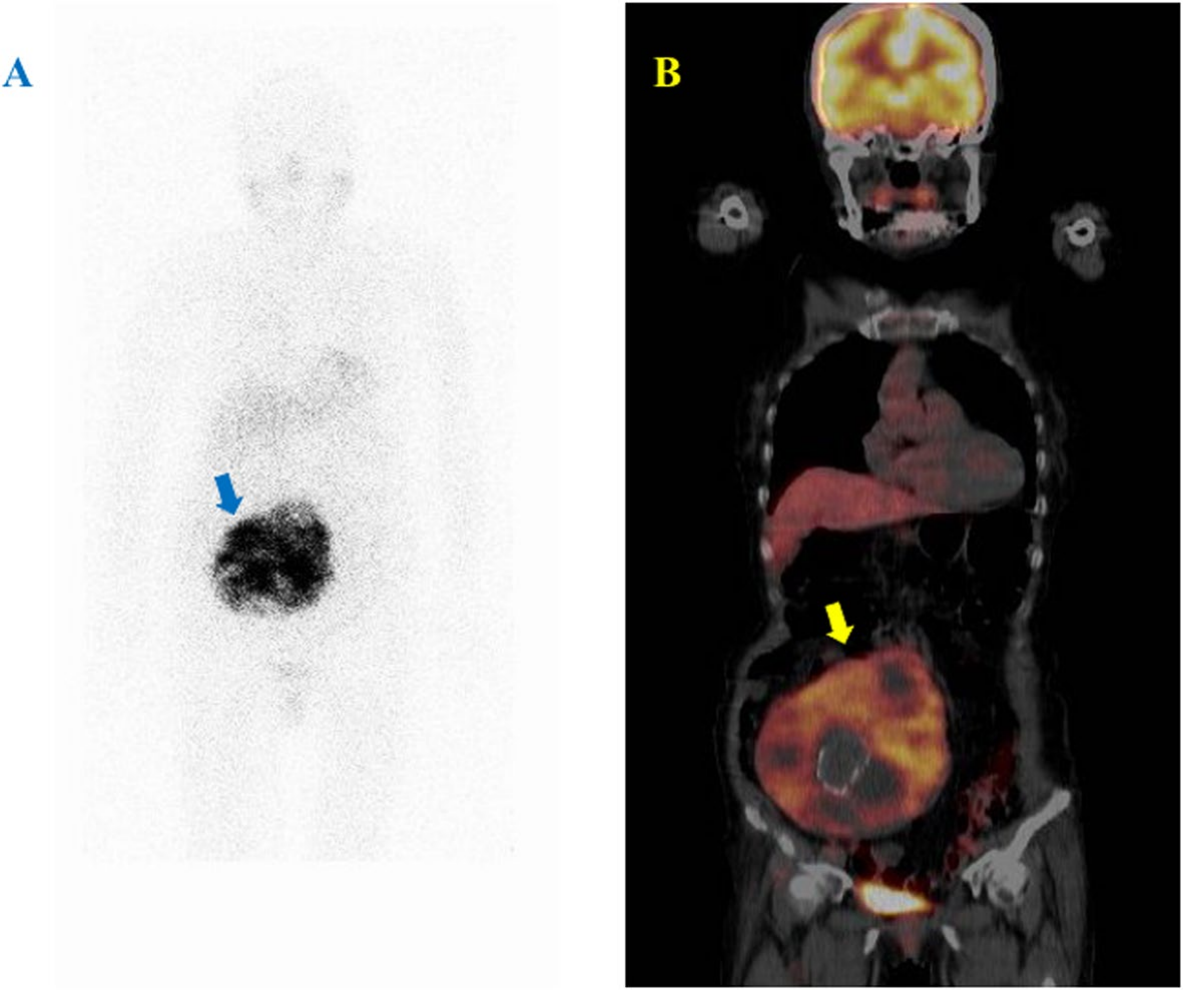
^18^F-FDG-PET and MIBG scintigraphy in paraganglioma without SDHB gene mutation. Sixty-one y.o. female of paraganglioma without SDHB gene mutation. (A) ^123^I-MIBG scintigraphy showed positive accumulation in right retroperitoneal to pelvic cavity. (B) ^18^F-FDG-PET showed SUVmax:5.0 in the mass. The tumor had PASS Score with < 4 and GAPP Score with well differentiation
